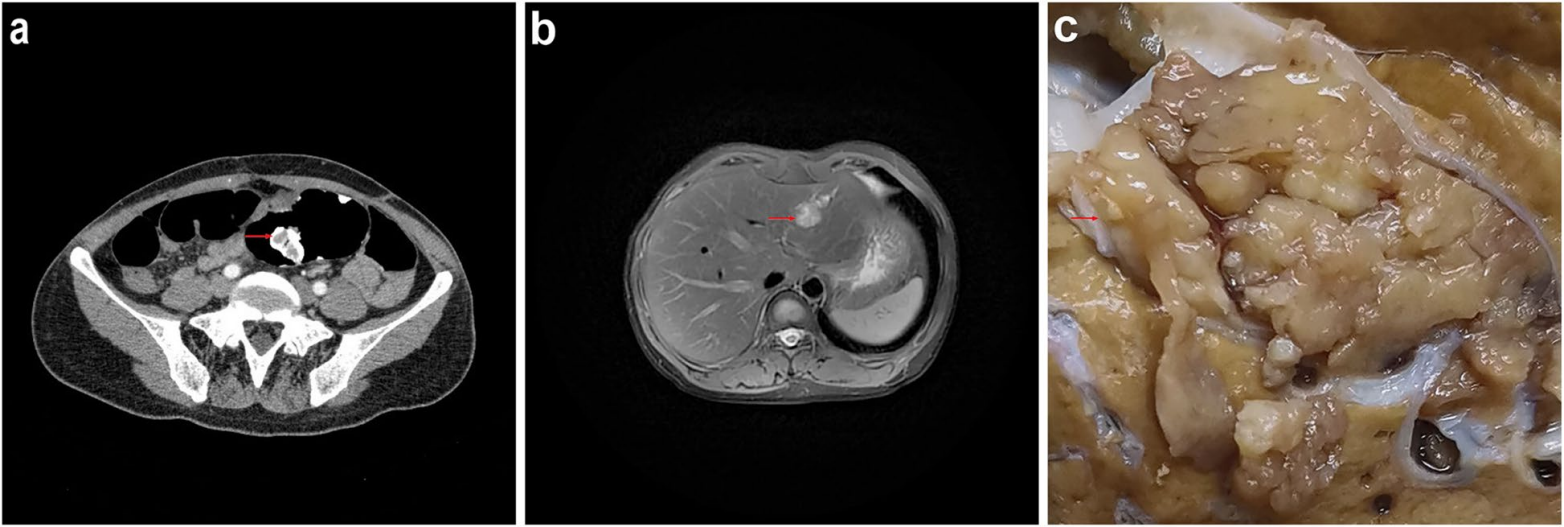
Pelvic CT and Abdominal MRI from the case and gross features of the neoplasm. a Pelvic CT showed nodular soft tissue shadow in the intestinal lumen of sigmoid colon, surrounded by high-density contrast agent. Red arrow indicates designate polyps. b Abdominal MRI showed a round low-density mass in the left lobe of liver with a clear border. Red arrow indicates designate IOPN. c Macroscopically, the neoplasm had a clear boundary with the surrounding liver tissue. Red arrow indicates designate IOPN
